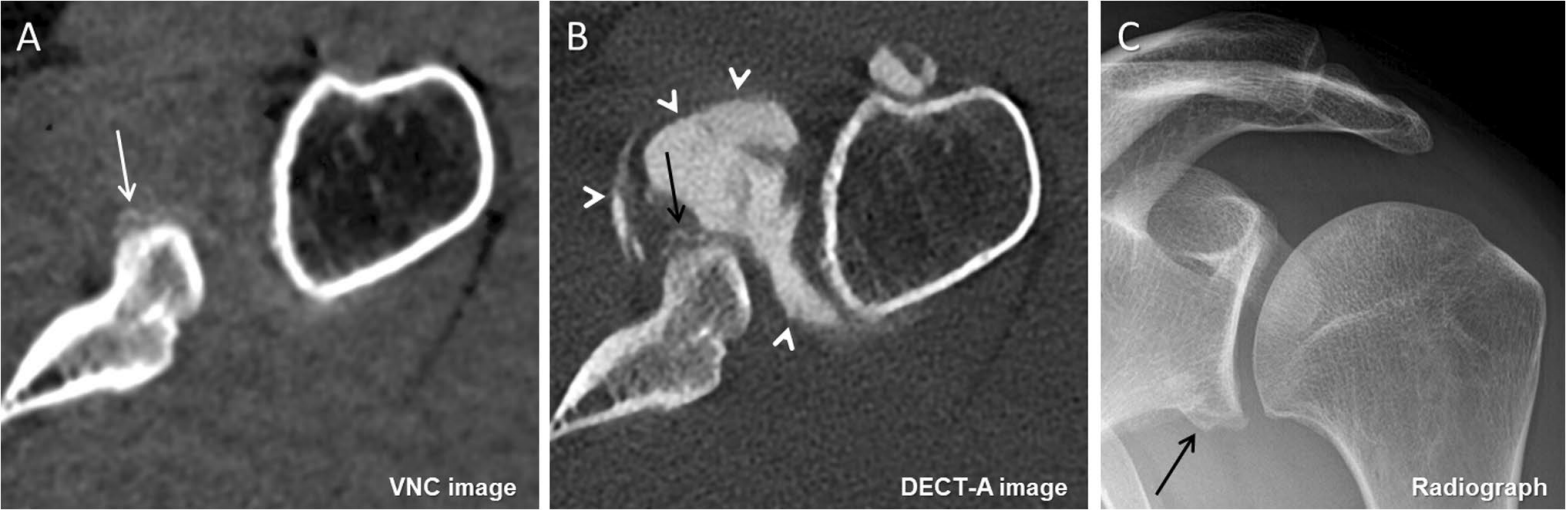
A 31-year-old male with recurrent anteroinferior shoulder dislocation. On the axial virtual non-contrast image (A), a thin periosteal calcification is visible (white arrow), which had been wrongly interpreted as labral-periosteal tear (black arrow) outlined by iodinated contrast material (arrowheads) on the axial DECT arthrography image (B). The coronal radiograph (C) confirms the shell-like periosteal calcification at the anteroinferior glenoid (arrow). The confidence level was high on image (A) and moderate on image (B)
